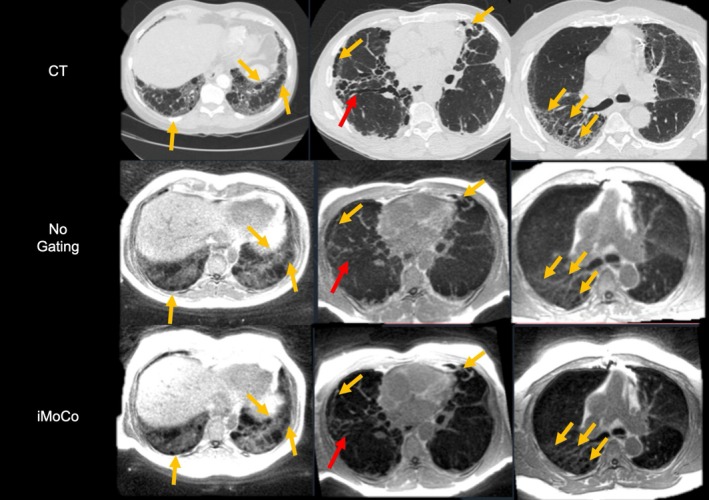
Improved visualization of clinically relevant parenchymal texture using iMoCo in patients with a diagnosis of IPF. Images of the lung parenchyma from three patients with patterns of “possible UIP,” “possible UIP,” and “definite UIP” are shown in columns A, B, and C respectively. Anatomically matched CT slices are shown in row 1, with corresponding UTE slices from no‐gating and iMoCo reconstructions in rows 2 and 3 respectively. Yellow arrows indicate locations of severe fibrotic changes distributed across the lung periphery, while the red arrow indicate airway structure, potentially suggesting traction bronchiectasis, visible on CT and iMoCo UTE, but obscured without motion compensation.
